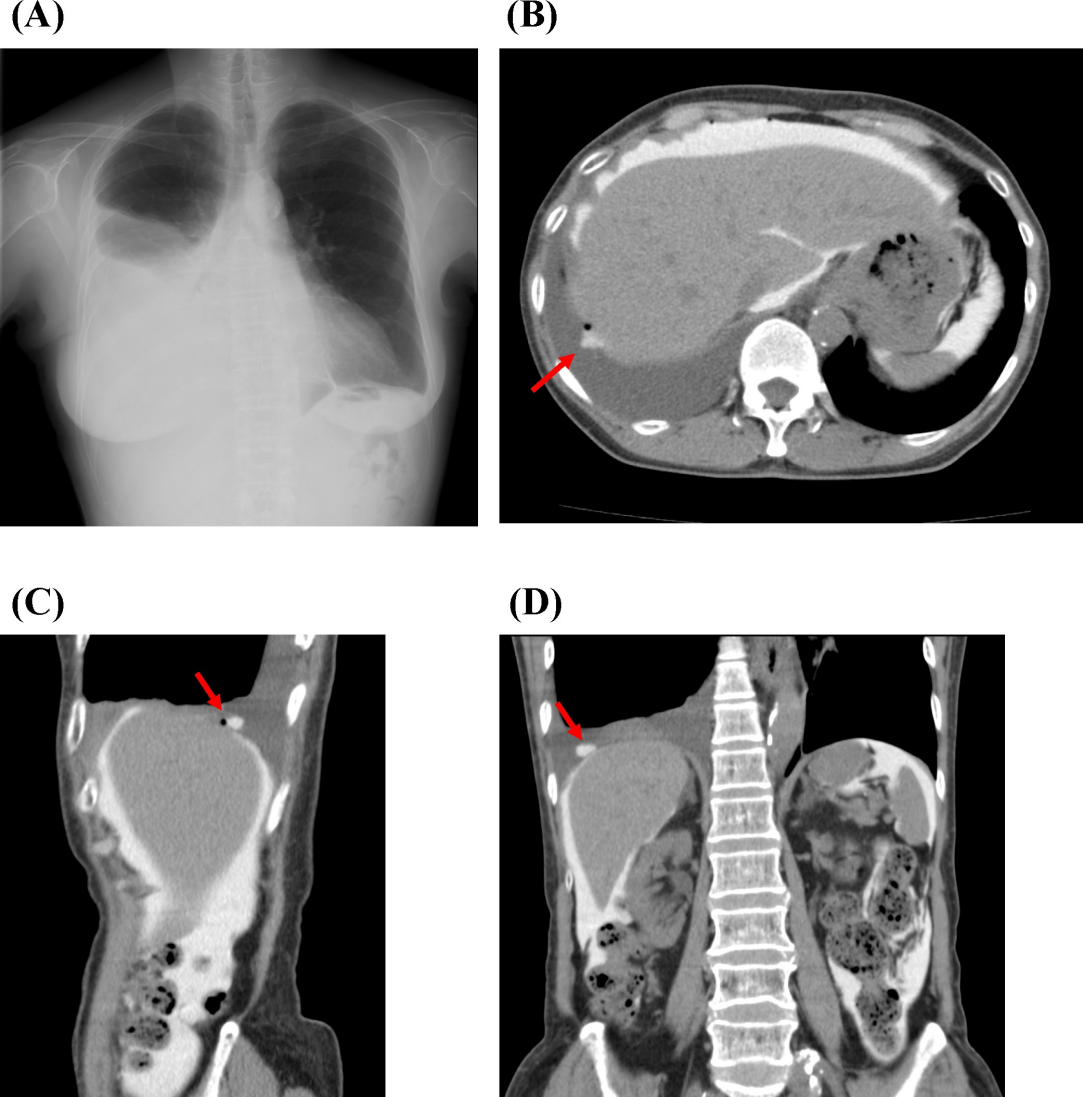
A case of hydrothorax in a peritoneal dialysis patient with a penetrated site. (A) Chest x-ray at the time of hydrothorax diagnosis. (B, C, D) Penetrated sites on the diaphragm as revealed by CT peritoneography on the transverse, sagittal, and coronal view, respectively (red arrows are penetrated sites).
